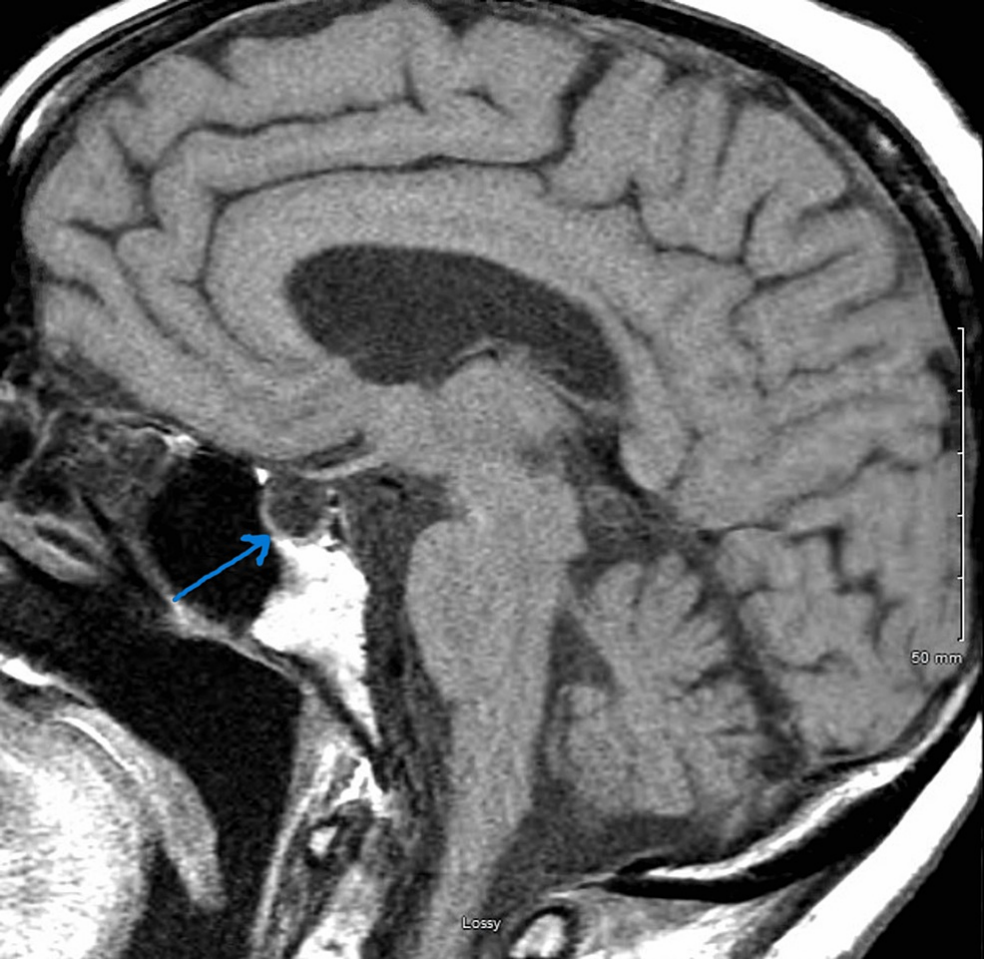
MRI showing empty sella.
